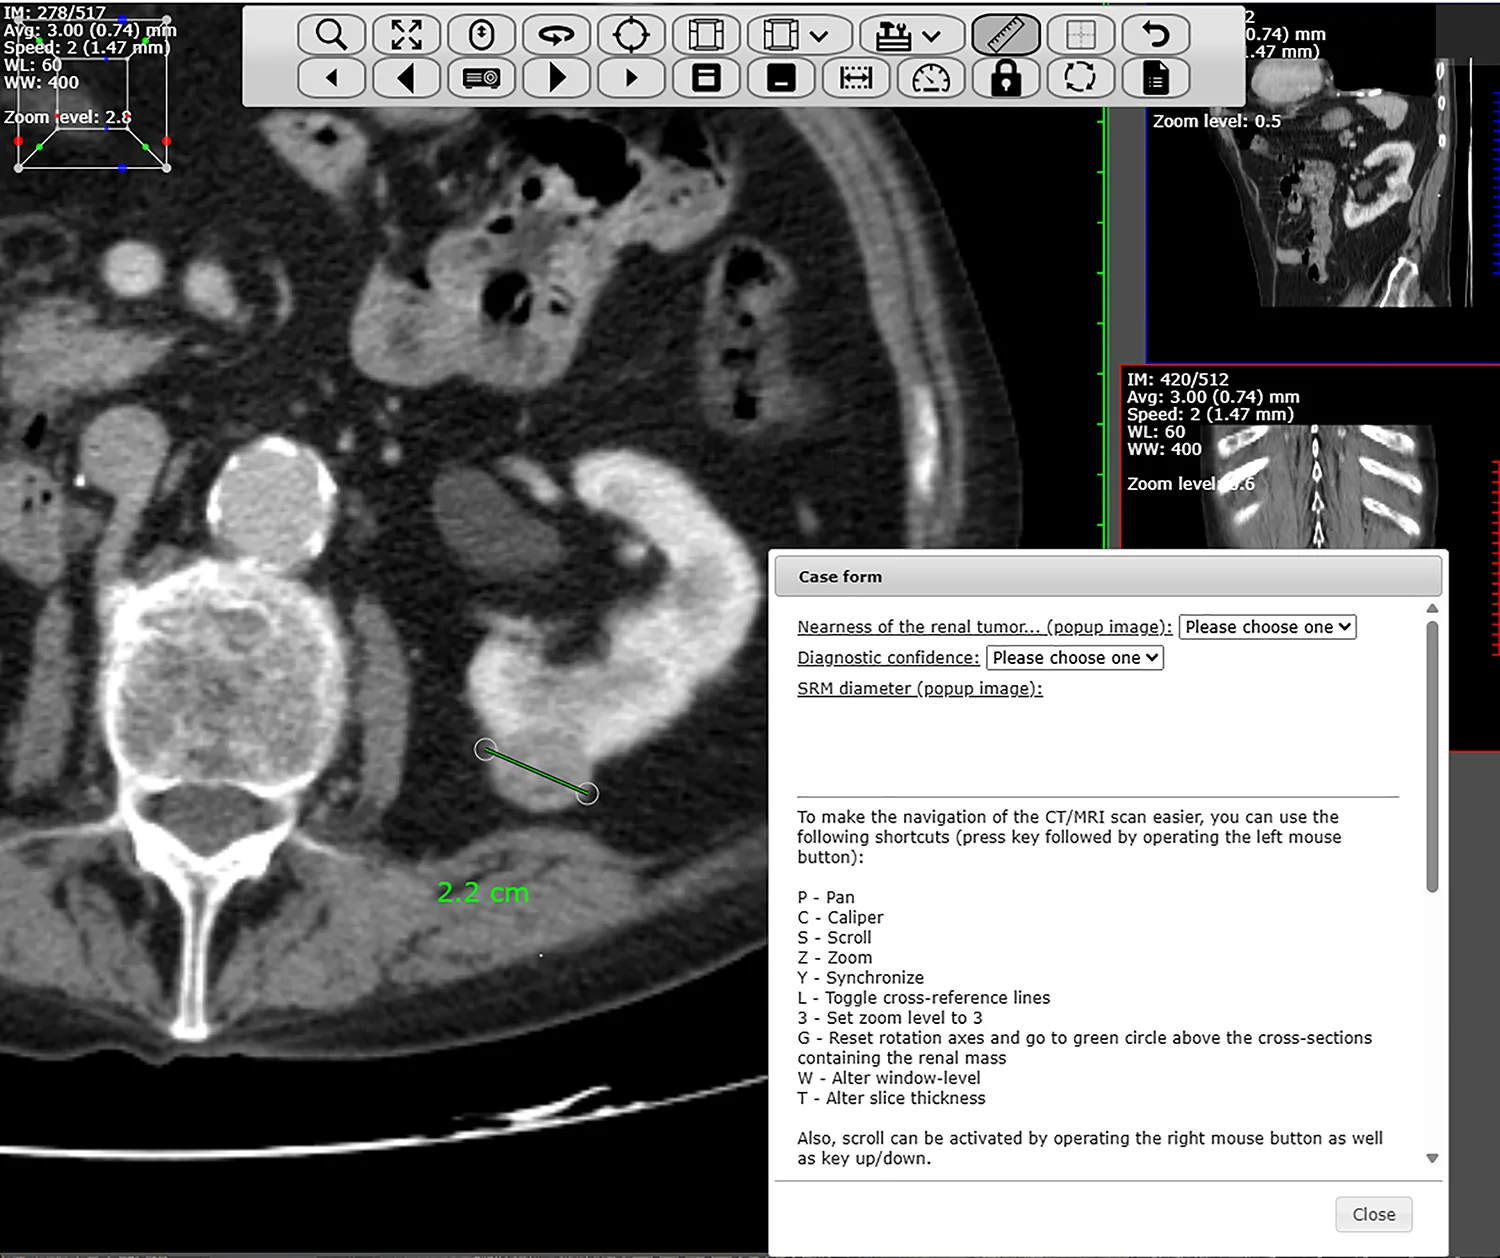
Screenshot of the web-based Digital Imaging and Communications in Medicine viewer equipped with a case report form. A 2.2 cm posterior exophytic small renal mass in the left kidney is depicted on a contrast-enhanced axial CT image accompanied by reconstructions in the coronal and sagittal planes
